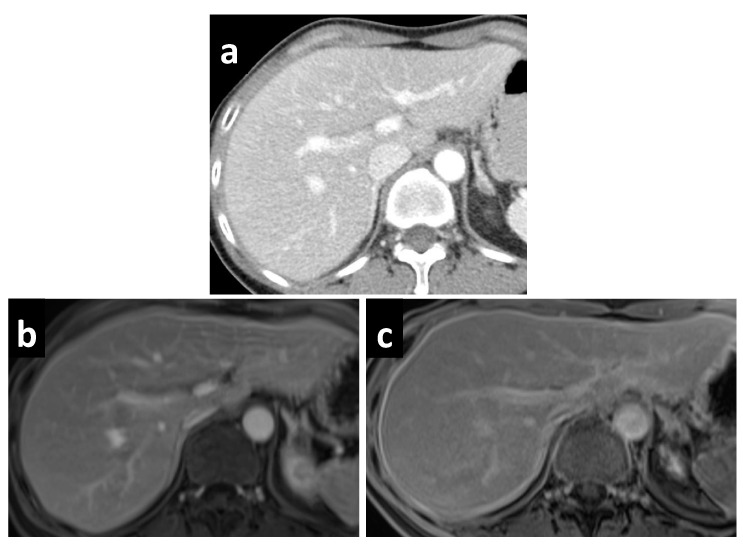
A 55-year-old female with PMP. (a) CT reveals no significant abnormality, but MRI performed on the same day demonstrates a thin enhancing line surrounding the liver on the (b) portal venous phase, which becomes more apparent on the (c) delayed phase.
